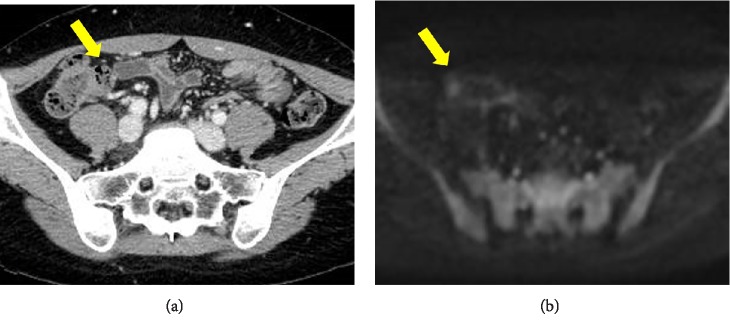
(a) CECT showing complete disappearance of the tumor at 2 months after steroid treatment (arrow). (b) MRI diffusion-weighted images indicate the absence of any lesion (arrow).
